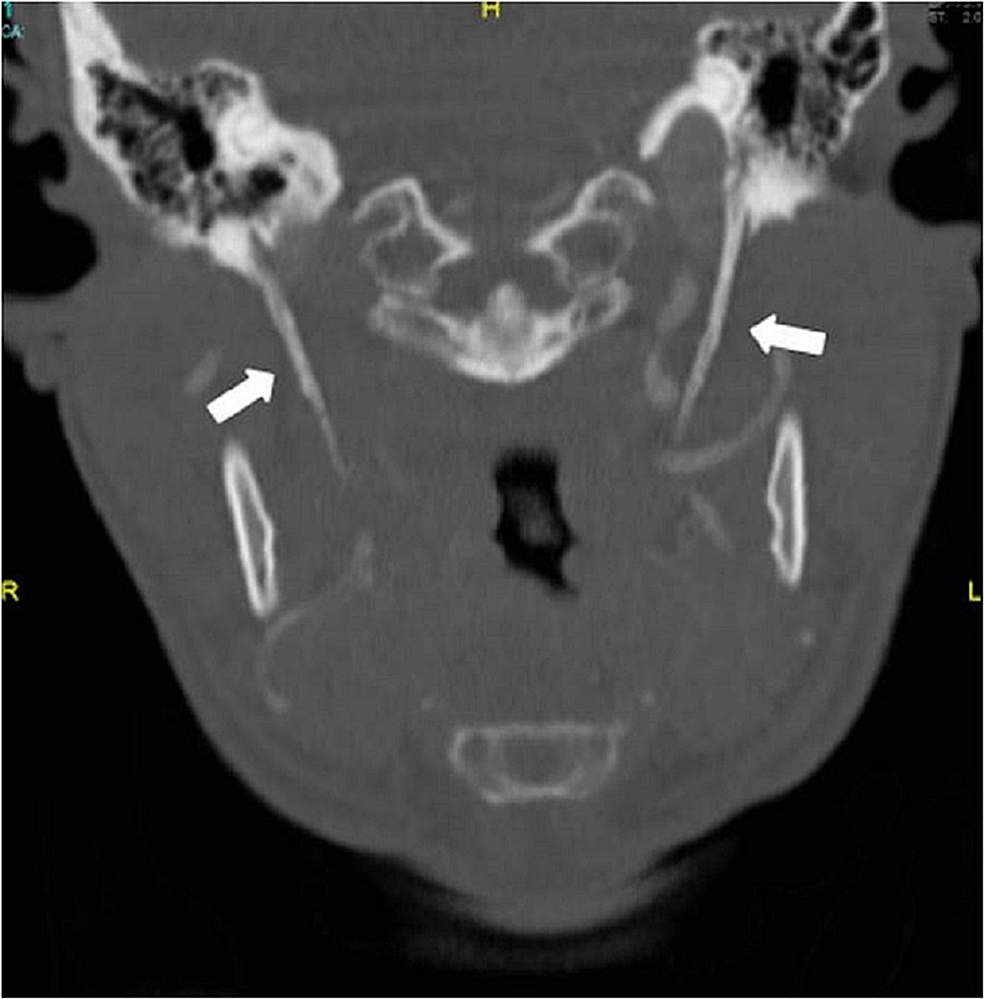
CT scan of the neck: coronal view. Arrows show the elongated styloid process. CT: computed tomography
